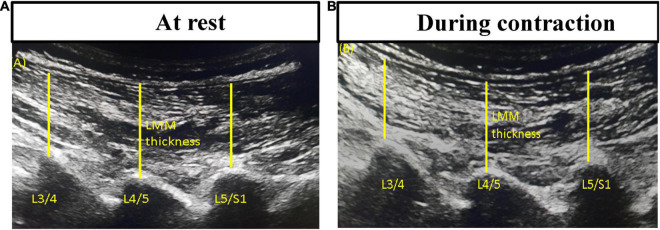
Thickness measurements of lumbar multifidus muscles using bright-mode ultrasound images (A) at rest and (B) during contraction.
